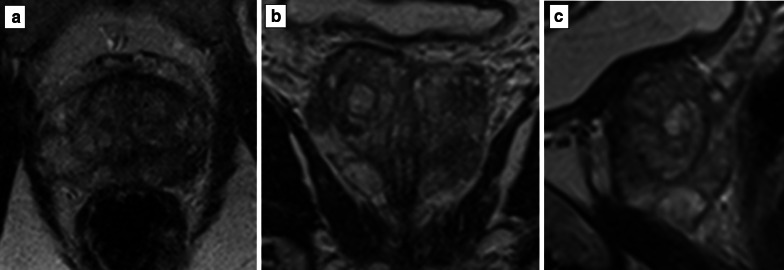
Axial (a), coronal (b) and sagittal (c) T2-weighted magnetic resonance imaging acquisitions of the same patient. Each acquisition was 4 min long, but the pixel size varied for each plane. The pixel size was 0.60 x 0.60 mm in (a), 0.67 x 0.67 mm in (b) and 0.80 x 0.80 mm in (c). This image shows that as the pixel size goes up, there is less noise but also that sharpness is reduced
